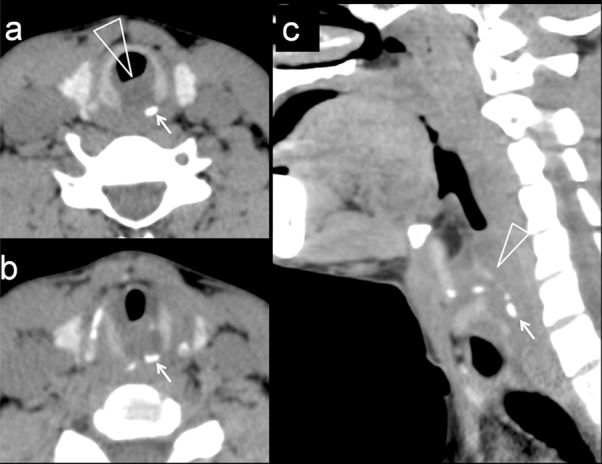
Axial non-contrast-enhanced CT image at the level of the cricoid cartilage (a) shows a discontinuous posterior ring with an isolated sclerotic component (arrow). There is diffuse soft tissue swelling, encroaching on the air column (triangle). (b) An additional axial image shows bone fragments (arrow). (c) Sagittal reconstruction demonstrates a hypodense postcricoid soft tissue swelling (triangle) with multiple bone fragments (arrow).
